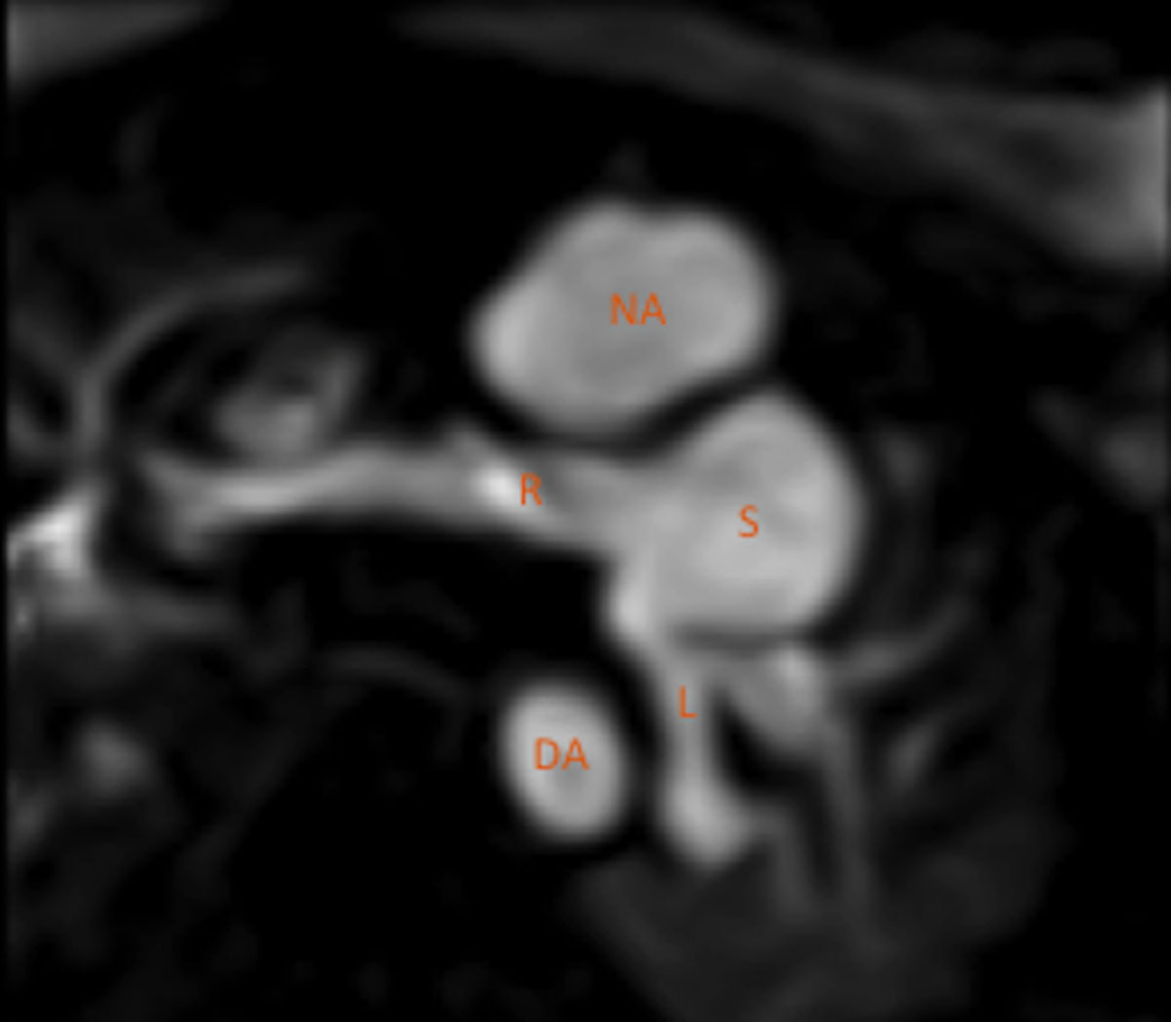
MRI Gadolinium enhanced image showing the PA confluence and stump positioned to the left of the DKS with LPA origin kinking. NA : Neo-Aorta. S: Stump. R: Right pulmonary artery. L: Left pulmonary artery. DA: Descending Aorta
